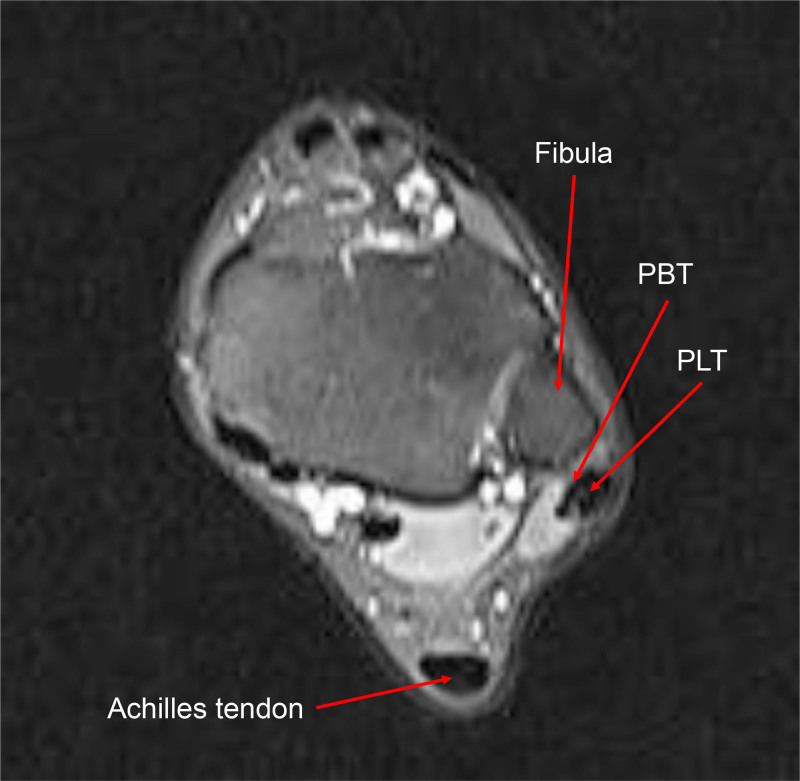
Preoperative MRI of the left ankle demonstrating peroneal tendon dislocation. The peroneus longus tendon, which normally lies posterolateral to the peroneus brevis tendon and posterior to the lateral malleolus, is laterally displaced over the fibula. MRI = magnetic resonance imaging, PBT = peroneus brevis tendon, PLT = peroneus longus tendon.
